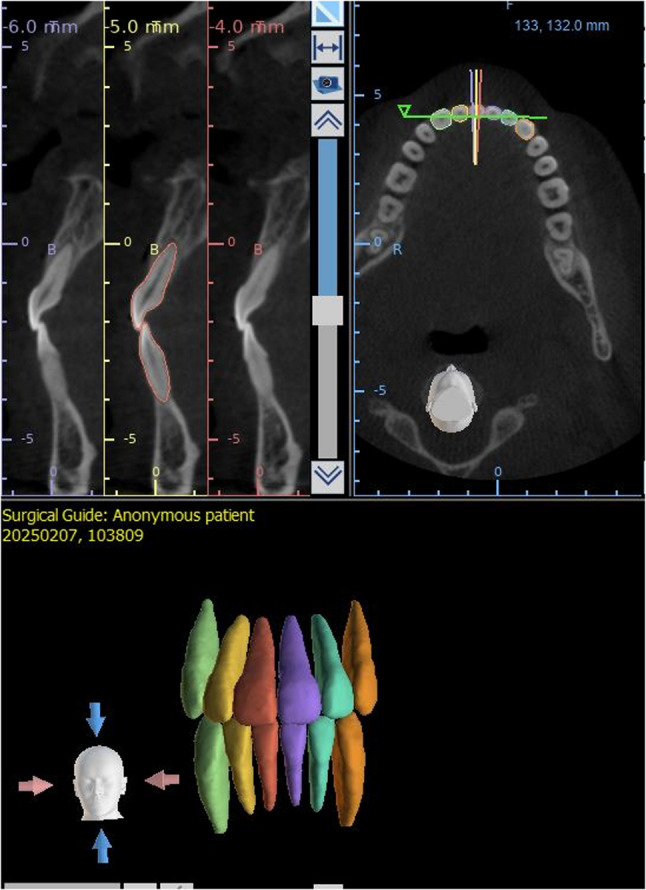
Radiographic evaluation of automatic teeth segmentation performed by Bluesky bio software
